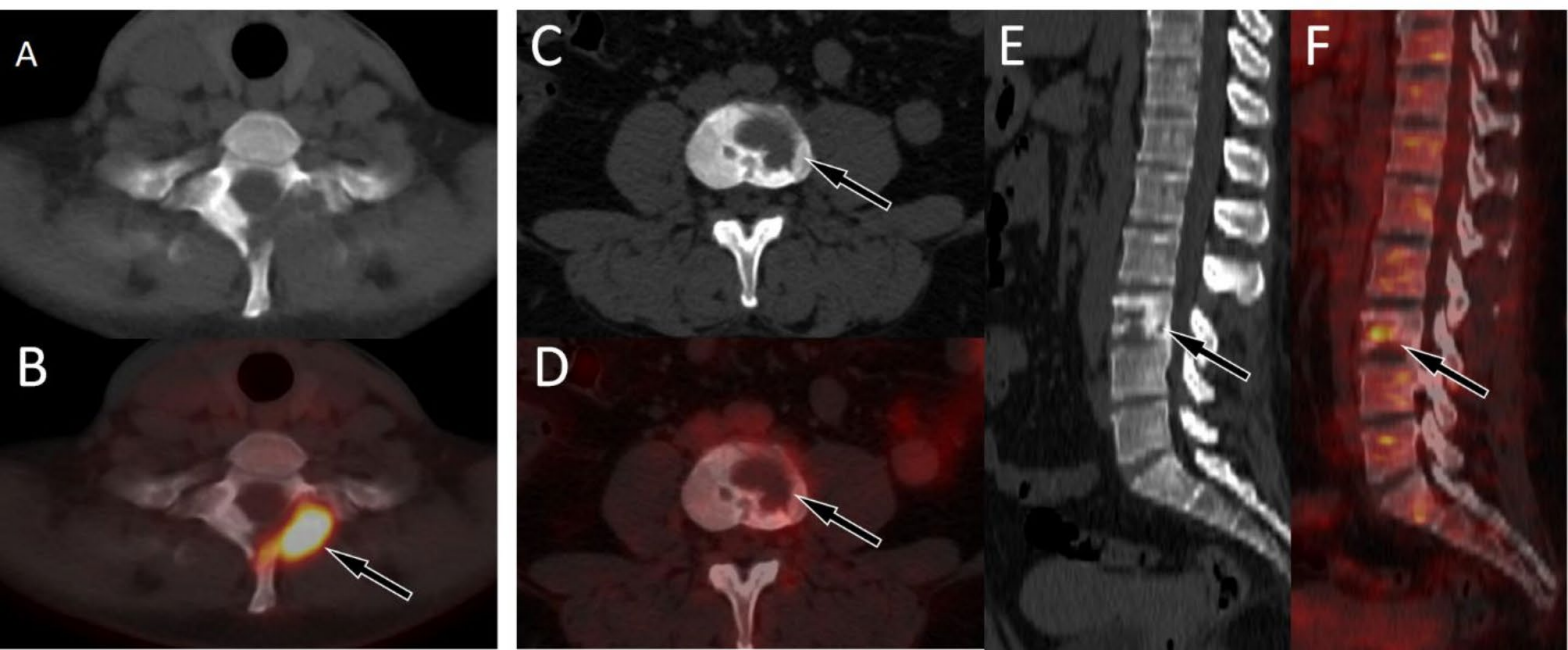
(A,B) A 37-year-old woman with LCH; (A) Axial CT reveals low-density osteolytic bone destruction shadow on the left vertebral plate of the first thoracic vertebra, without sclerotic rims (arrow); (B) The PET/CT fusion image of the corresponding area shows obviously increased ^18^F-FDG uptake (arrow), with a SUVmax of 8.9, suggesting that the lesion is in an active phase. (C–F) A 23-year-old woman with LCH; (C), Axial CT revealed low-density osteolytic bone destruction in the third lumbar vertebrae (arrow), without the formation of soft tissue masses; (D), The PET/CT fusion image of the corresponding area shows mildly increased ^18^F-FDG uptake (arrow), with a SUVmax of 3.5; and sagittal images (E CT; F PET/CT) revealed lesions with osteosclerotic rims (arrows), suggesting that the lesion may be in remission. LCH = langerhans cell histiocytosis.
